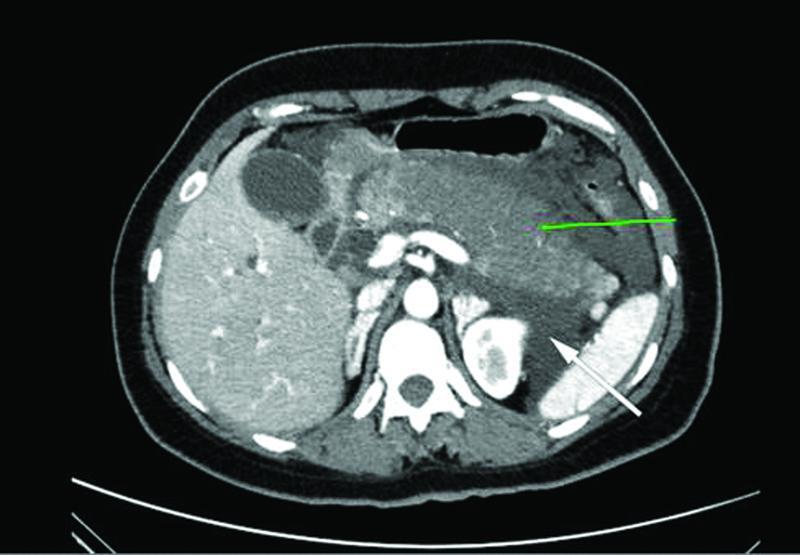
Computed tomography scan image showing acute necrotizing pancreatitis involving the body and tail of the pancreas (green arrow), with acute peripancreatic fluid collections (white arrow).
